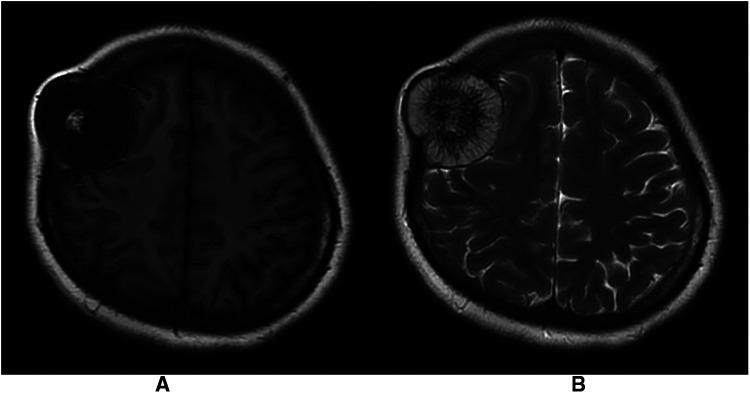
(A) Mixed long T1 signal shadow with irregular short T1 signal shadow in T1-weighted images. (B) Mixed long T2 signal shadow with radiolucent short T2 signal shadow in T1-weighted images.
